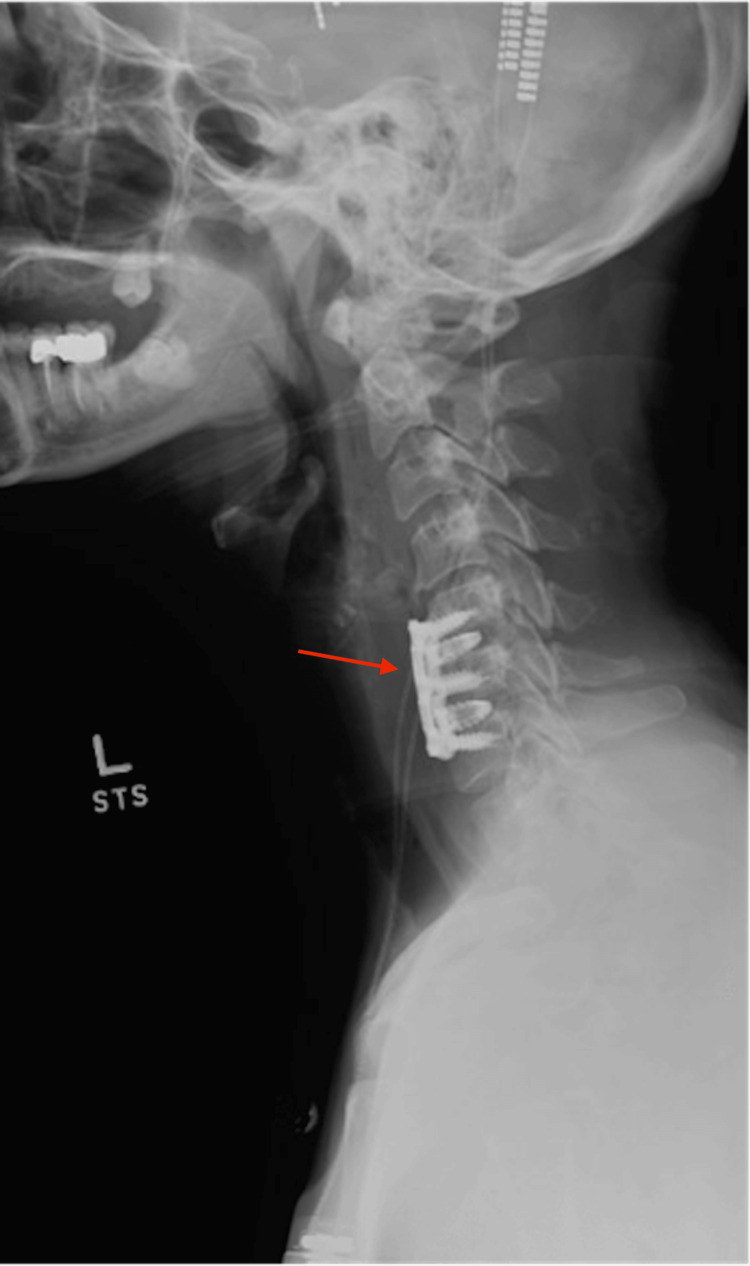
X-ray of the neck shows the placement of anterior cervical discectomy and fusion hardware with no loosening or malalignment
